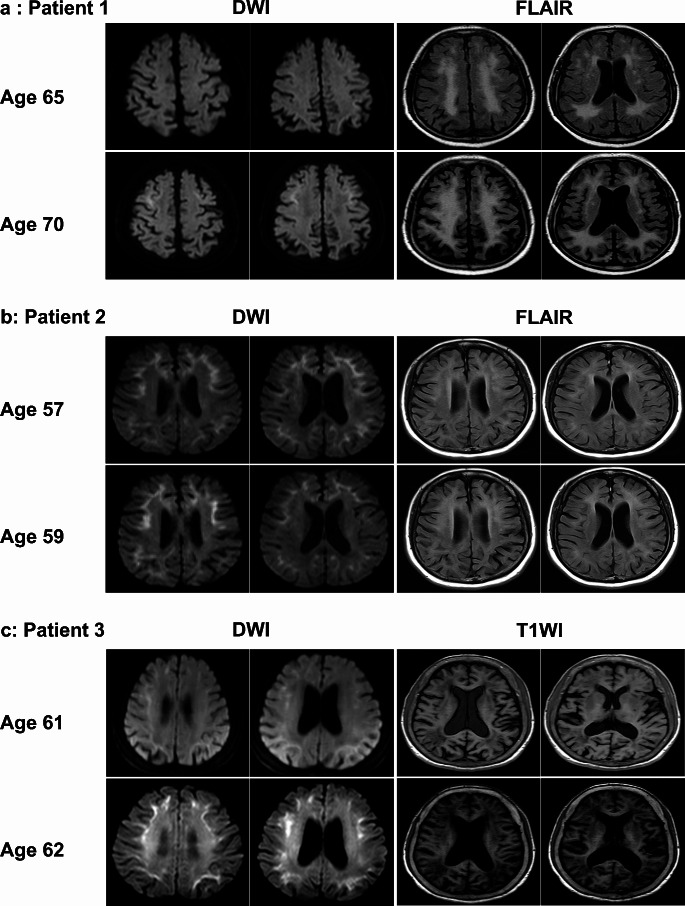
Dynamic brain imaging changes in three patients with neuronal intranuclear inclusion disease (NIID). Patient 1 (a) with NIID showed typical DWI hyperintensity in the bilateral frontal corticomedullary junction area at five-year follow-up, with further extension of white matter hyperintensity on FLAIR. On DWI, the high signal in the left temporal lobe disappeared after two years in patient 2 (b). Serial MR images of patient 3 (c) show an anterior to posterior propagation pattern of curvilinear hyperintense signals on DWI and apparent cerebral atrophy on T1WI
